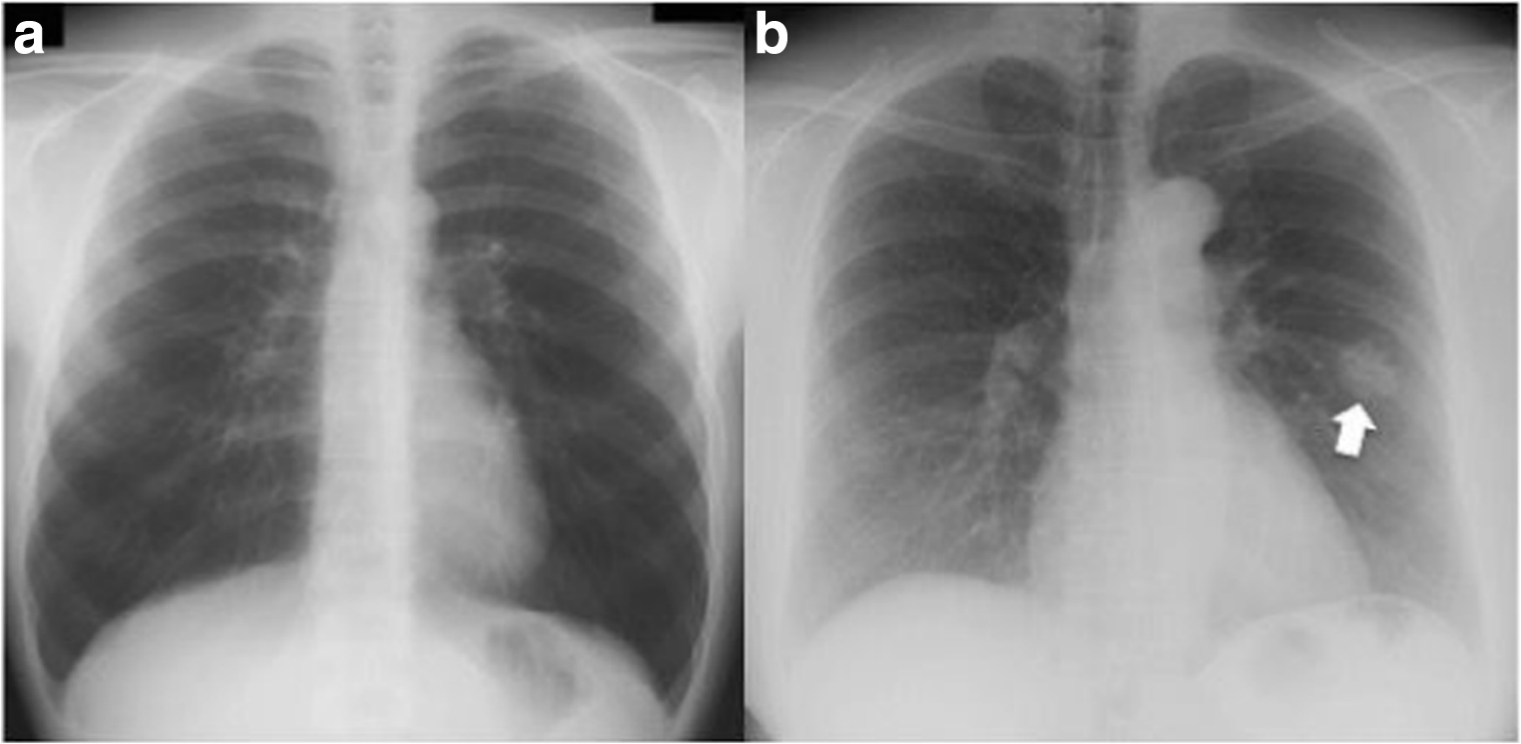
Exemplars from the stimuli sets. (a) Normal chest radiograph presented in the chest priors task; (b) nodule radiograph presented in the nodule task (indicated by the white arrow, not present in the actual displays)
